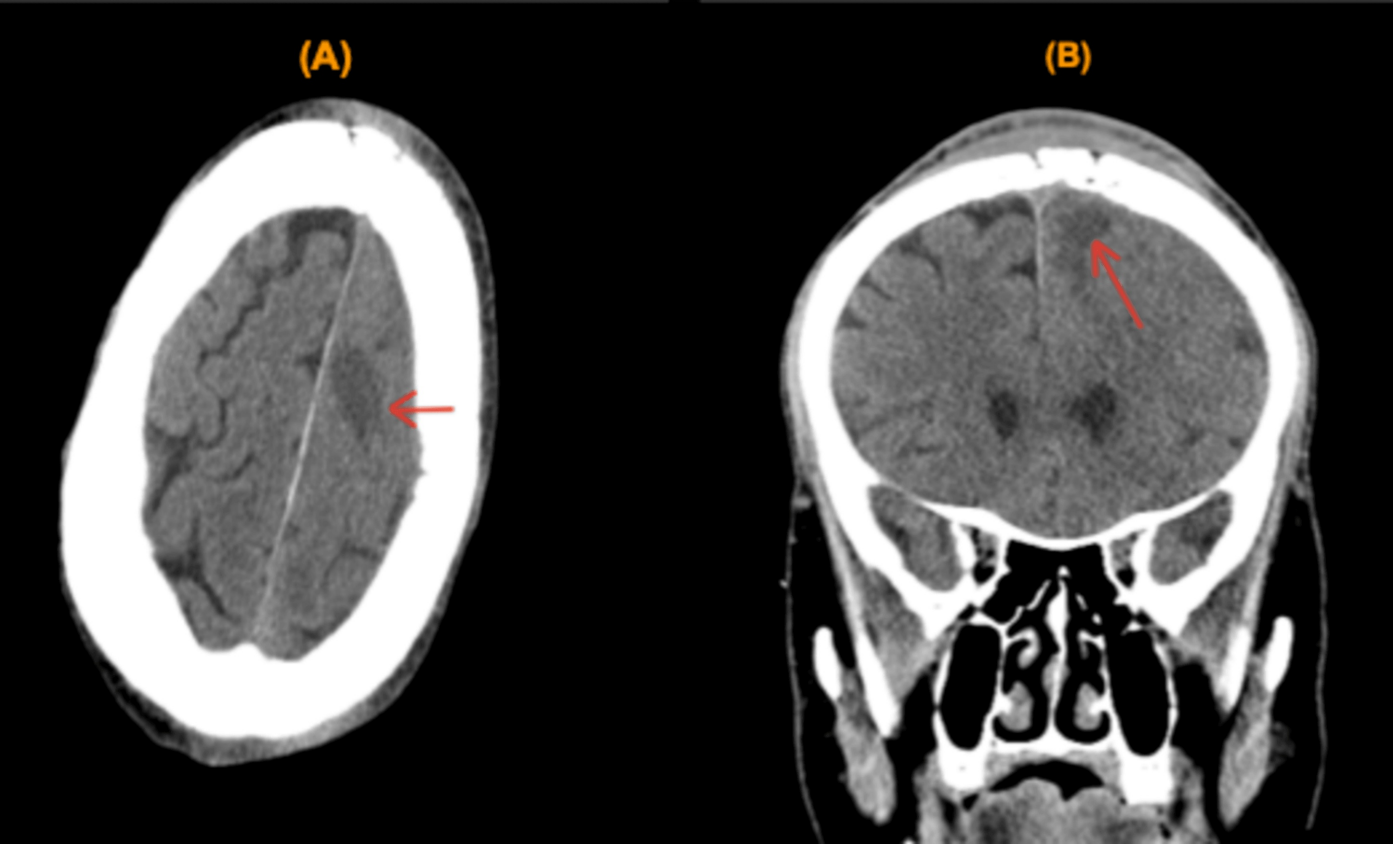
CT scan of the head. (A) Axial plane. (B) Coronal plane. CT scan of the head. (A) Axial plane. (B) Coronal plane. The red arrow points to a lytic lesion near the frontal bone with a midline shift.
